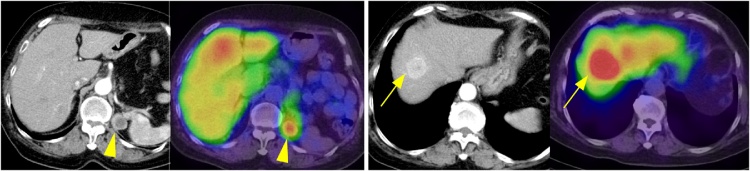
Contrast-enhanced CT and ^123^I MIBG scintigraphy findings. CT image showing ring-enhanced tumors in the left retroperitoneal area (arrowhead) and liver (arrow). Uptake of metaiodobenzylguanidine (^123^I-MIBG) in these tumors was confirmed during scintigraphy (arrow). CT, computed tomography.
